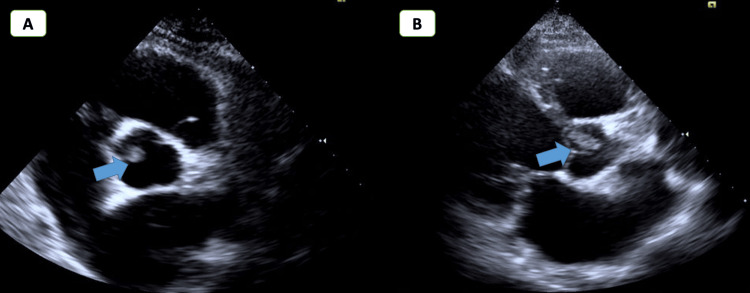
TTE with short and long parasternal axis view showing a mass attached to the aortic valve TTE: Transthoracic echocardiography
